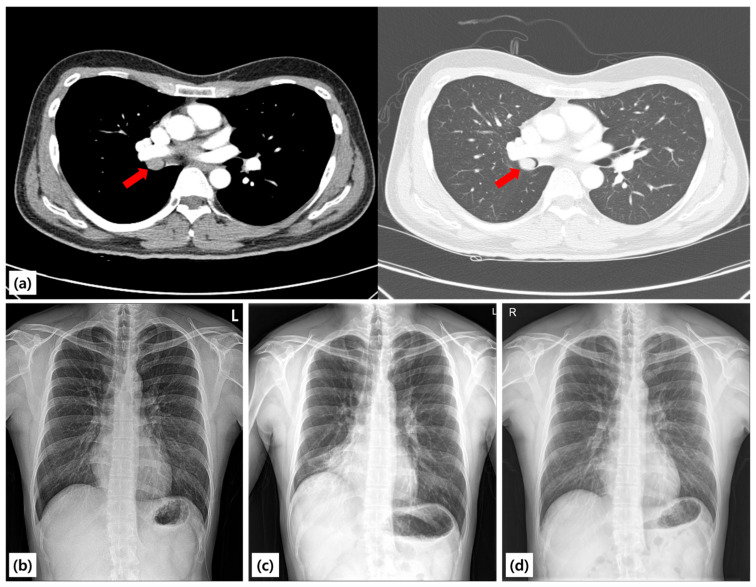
Chest radiographs and CT findings. (a) Pre-procedure chest CT scan showing a mass completely obstructing the proximal bronchus intermedius with distal atelectasis (red arrow). (b) Pre-procedure chest radiograph demonstrating volume loss of the right middle and lower lobes, manifested by elevation of the right hemidiaphragm and increased opacity in the right lower lung field, consistent with atelectasis. (c) Chest radiograph obtained two years earlier showing normal findings. (d) Post-procedure chest radiograph showing normalization of lung volume and resolution of atelectasis.
